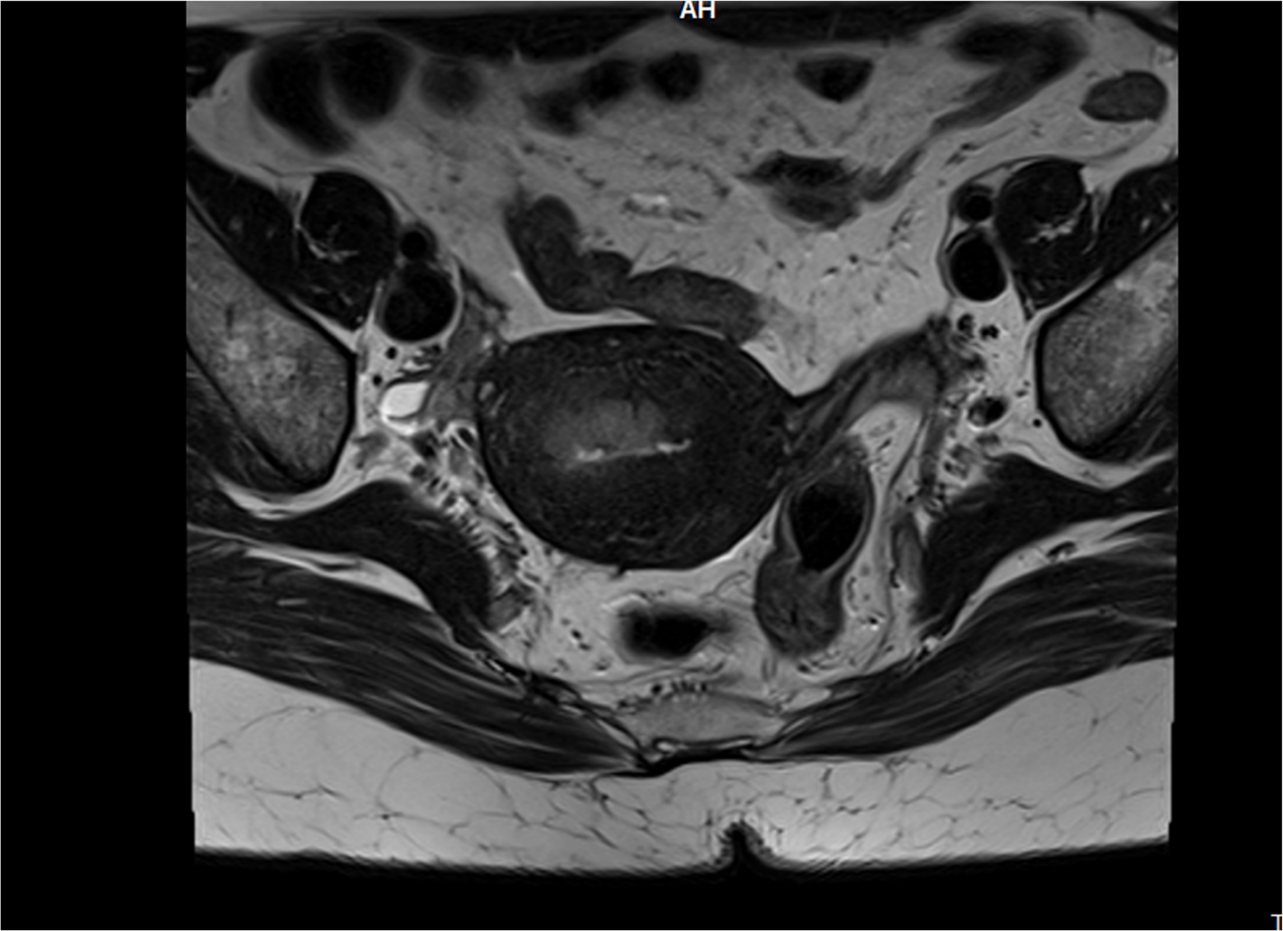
MRI showing enhancing lesion in the endometrium extending into the myometrium
